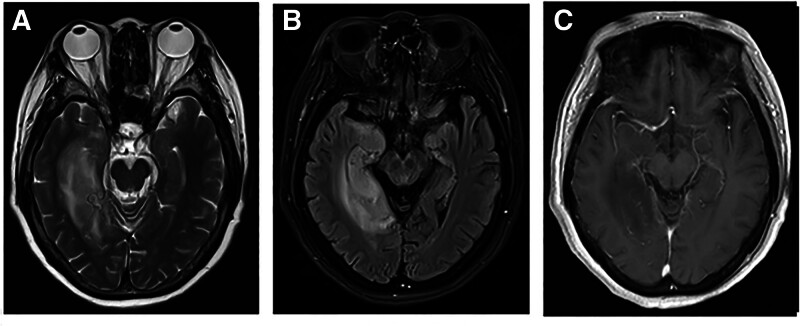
Baseline cranial MRI scan features of the patient before treatment. (A) The abnormal hyperintensity and swelling in the medial aspect of the right temporal-occipital lobe on T2-weighted sequence. (B) The abnormal hyperintensity and swelling in the medial aspect of the right temporal-occipital lobe on FLAIR-weighted sequence. (C) The lesions in the medial aspect of the right temporal-occipital lobe exhibit no contrast enhancement.
